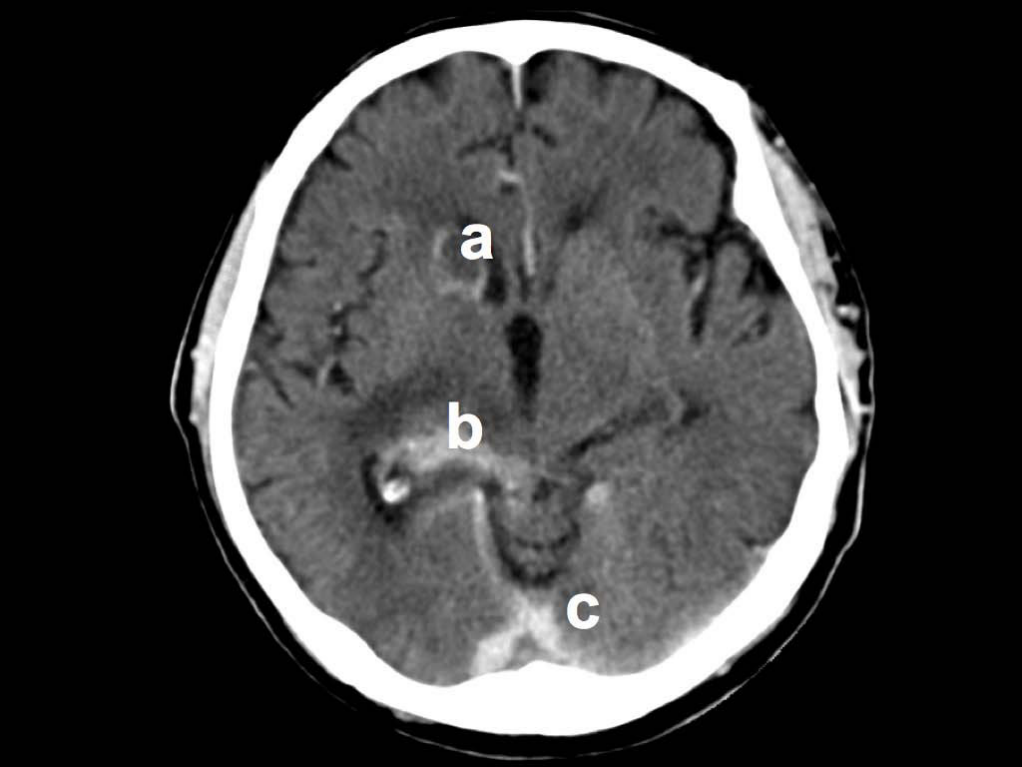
Axial computed tomography scan of the brain at day nine after presentation. There are contrast enhancement of (a) the right caudate nucleus, (b) the right medial geniculate nucleus and thalamus, and (c) the cerebellar tentorium. These enhancements are consistent with tuberculomas and leptomeningeal infiltration by *Mycobacterium tuberculosis*.
